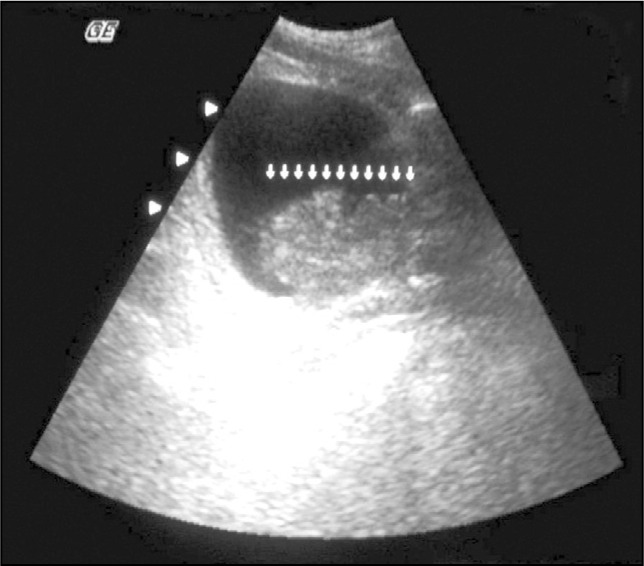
Abdominal ultrasound showing intravesical mass (10 cm in diameter).
